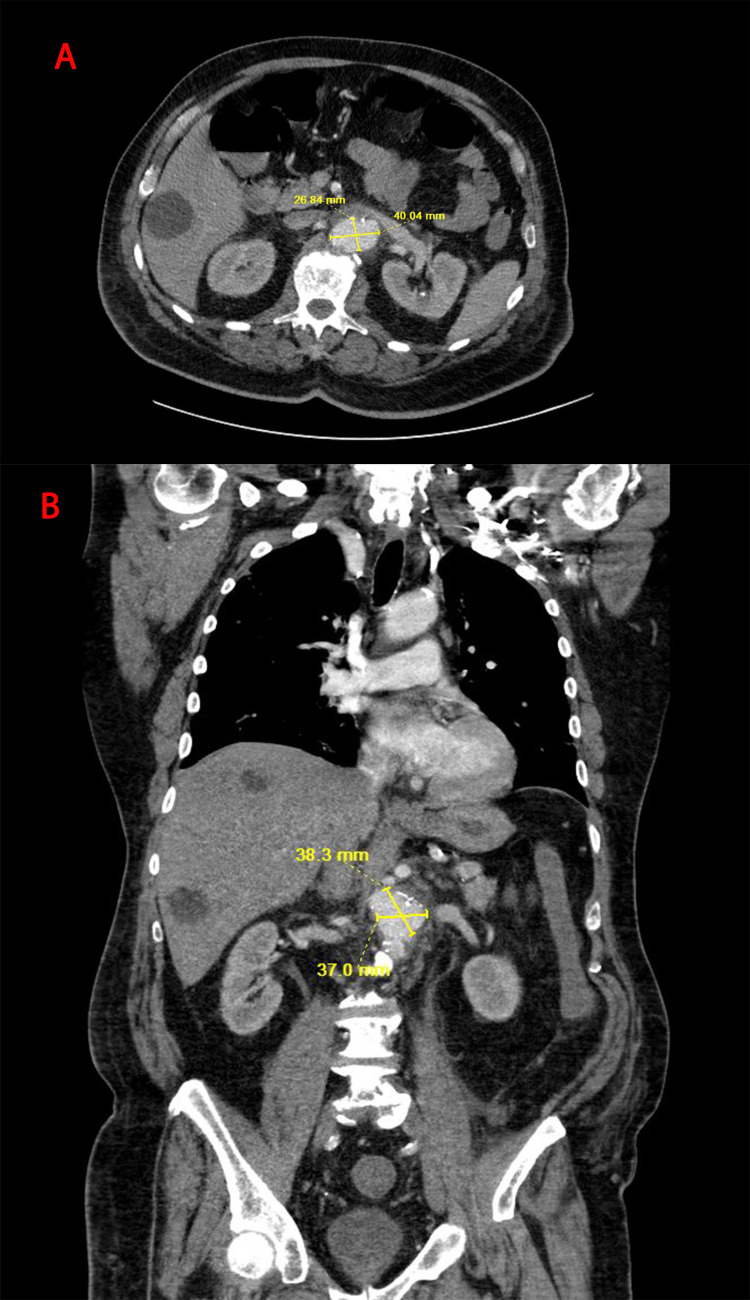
Computed tomography scan of the abdomen and pelvic Computed tomography scan of the abdomen and pelvic at hospital day six showed a new around 4 x 3 cm saccular aortic aneurysm from the left aspect of the proximal abdominal aorta. A: Axial section. B: Coronal section
